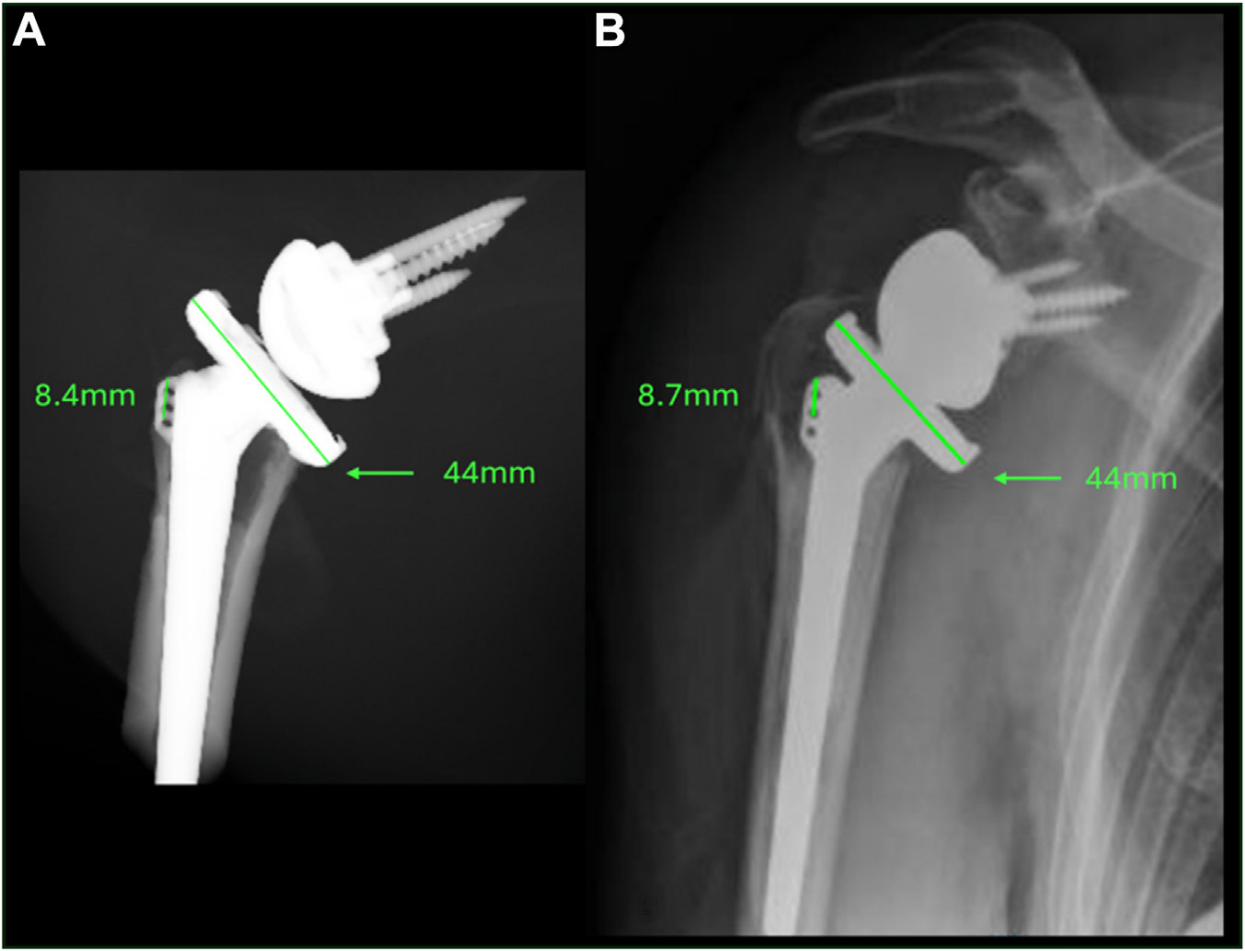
(A) The digitally reconstructed radiograph created using the VSP at the same angle as the postoperative radiograph. (B) The postoperative radiograph in AP direction, calibrated for measurements using the size of the prosthesis (*right*). The humeral tray was used for calibration (44 mm). The difference in planned and realized stem height is 0.3 mm (measured from proximal stem suture holes to medial calcar plane). *AP*, anteroposterior; *VSP*, virtual surgical planning.
